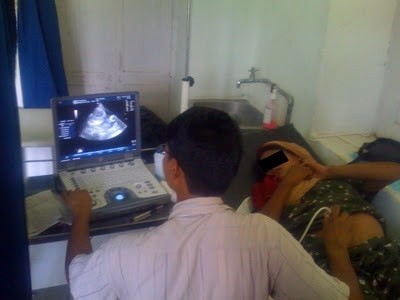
Nyaya Health physician Dr. Jhapat Thapa performing ultrasound on a pregnant patient.
